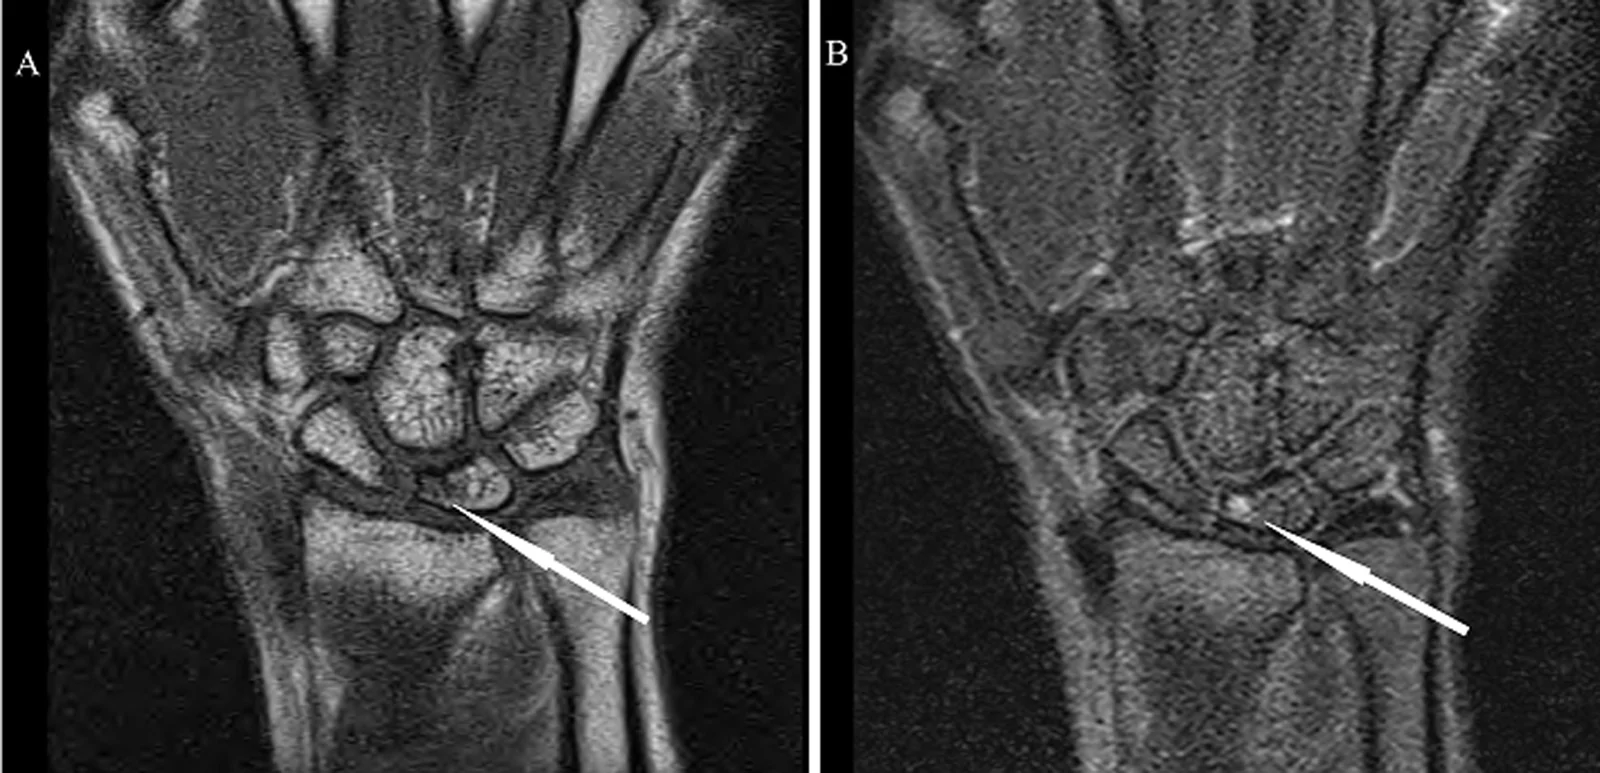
A) coronal T1-weighted MRI of the wrist demonstrates a focal hypointense marrow signal in the lunate (arrow), suggestive of early ischemic change; B) coronal proton density fat-saturated (PD-FS) MRI shows a corresponding focal hyperintense signal in the lunate (arrow), consistent with marrow edema, raising suspicion for early avascular necrosis
